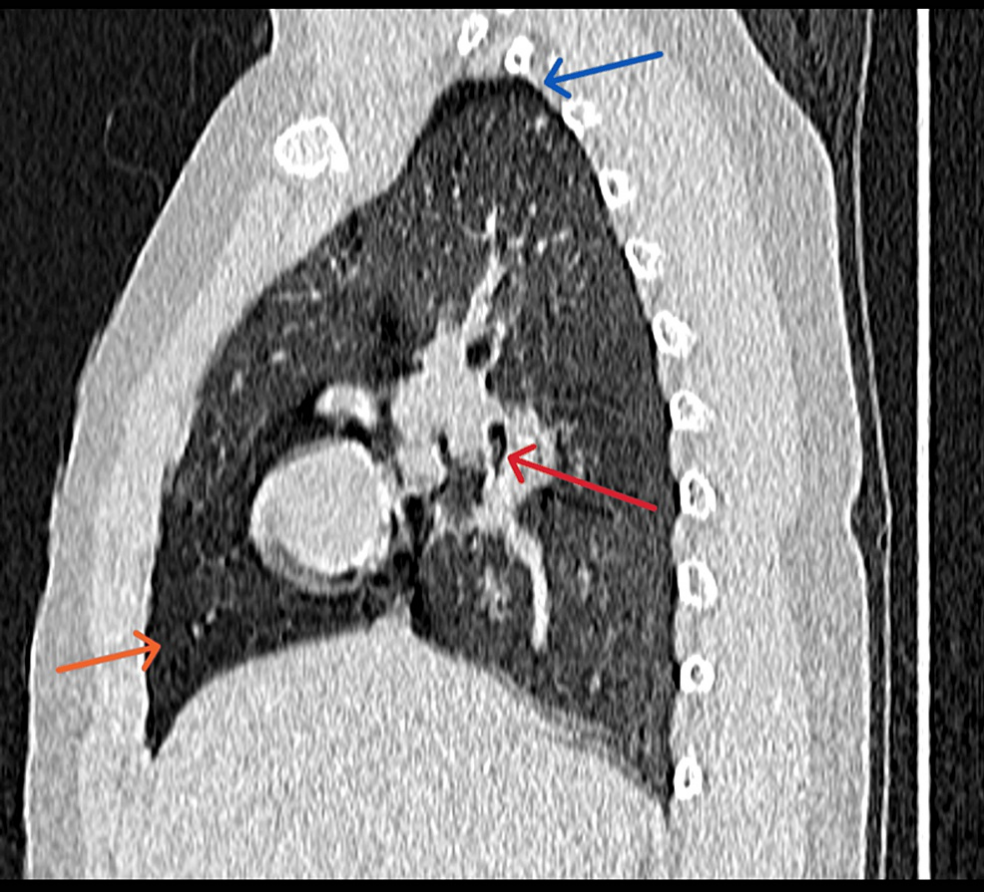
A slice of CT (sagittal view) showed the foreign body (red arrow), pneumothorax, pneumomediastinum (blue arrow), and middle lobe air trapping (orange arrow).
